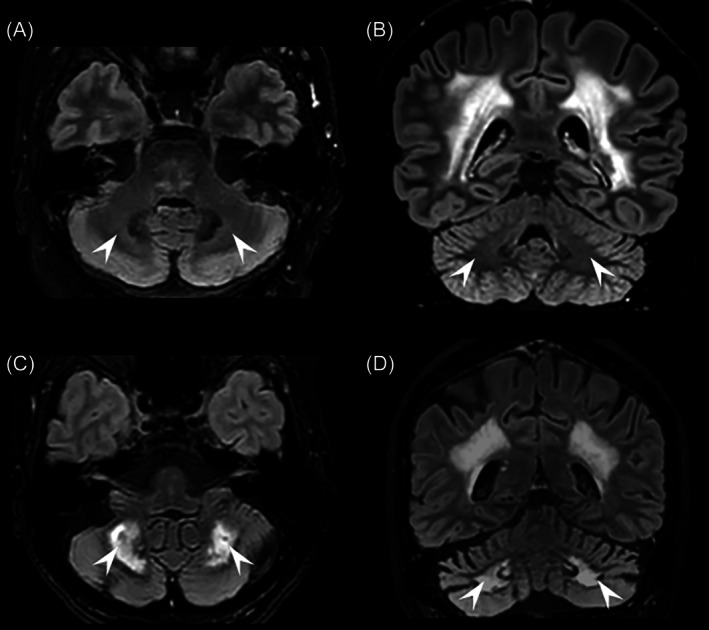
Cerebellar brain magnetic resonance imaging (MRI), fluid‐attenuated inversion recovery (FLAIR) images: (A) axial image in a 68‐year‐old *SLC20A2* female showing mild cerebellar hemispheric leukopathy; (B) coronal image in a 31‐year‐old *MYORG* male (A, B) with mild cerebellar hemispheric leukopathy; severe supratentorial band‐like leukopathy was also recognizable; (C, D) axial and coronal images in a 71‐year‐old *MYORG* female revealing severe cerebellar hemispheric leukopathy with subcortical white matter sparing; severe supratentorial band‐like leukopathy is also evident in (D).
